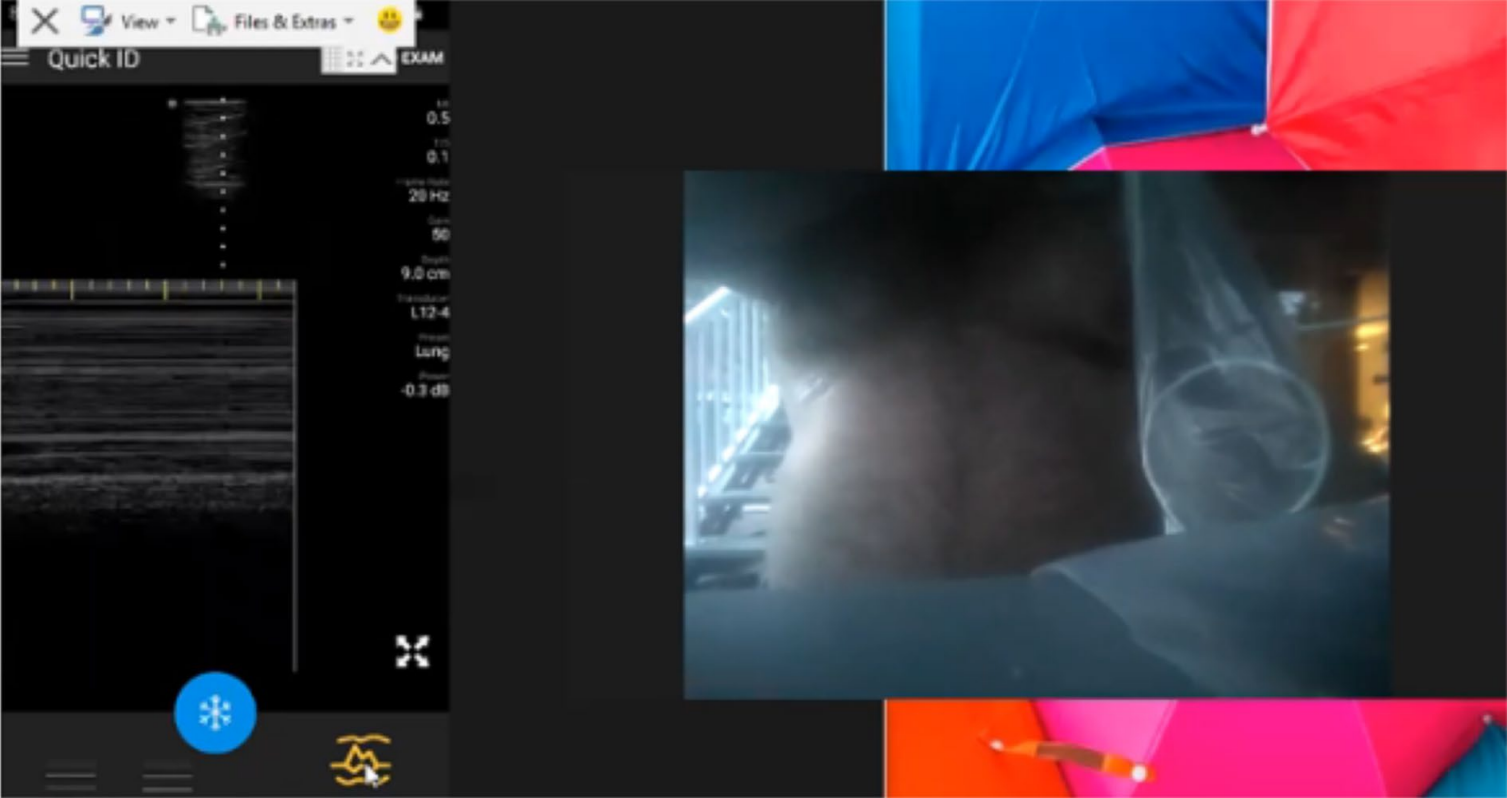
Baseline Self-Performed Lung Ultrasound Study of 32-year-old patient 6 months prior to COVID-19 diagnosis. Screenshot of the Remote Mentors Computer Screen that shows the patient imaging himself to the right of the screen and the resultant ultrasound image showing the “seashore” sign to the left. As lung ultrasound is a dynamic real-time examination hard to capture in any single image, readers are strongly encouraged to view the accompanying supplemental videos of the entire examination online to gain a true appreciation of the technique
